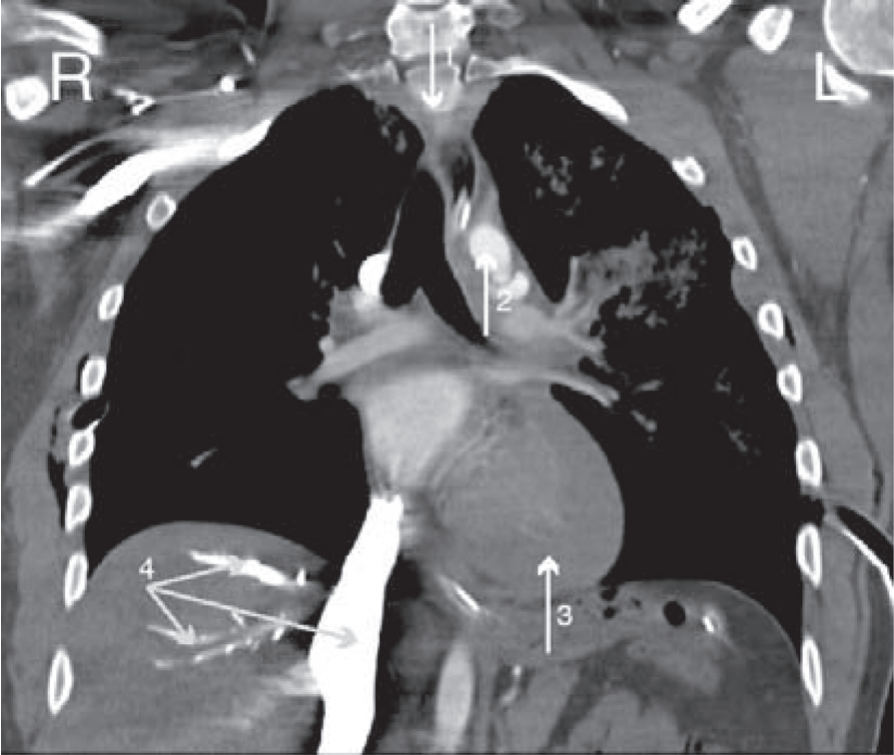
Coronal contrast CT chest showed mild right sided deviation of the trachea (1), pseudoaneurysm of the descending aorta (2), hemopericardium (3) and contrast reflux into the IVC and hepatic veins (4)
